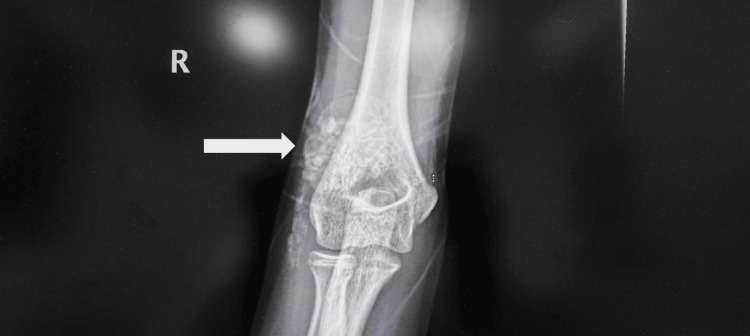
Anteroposterior (AP) X-ray of right elbow shows right elbow periarticular calcification (arrow).
